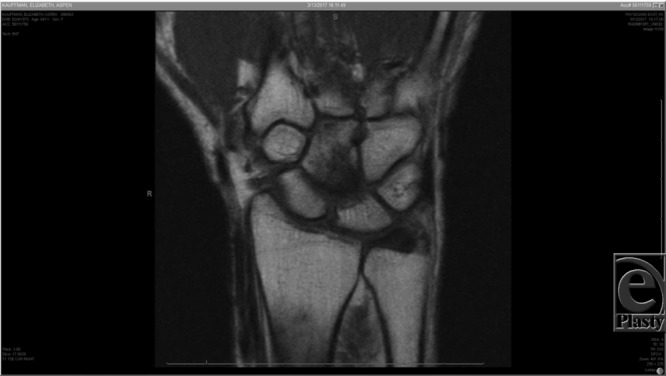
Coronal MRI slice of the right wrist. The proximal pole of the capitate has a low-intensity signal (Milliez type I).
